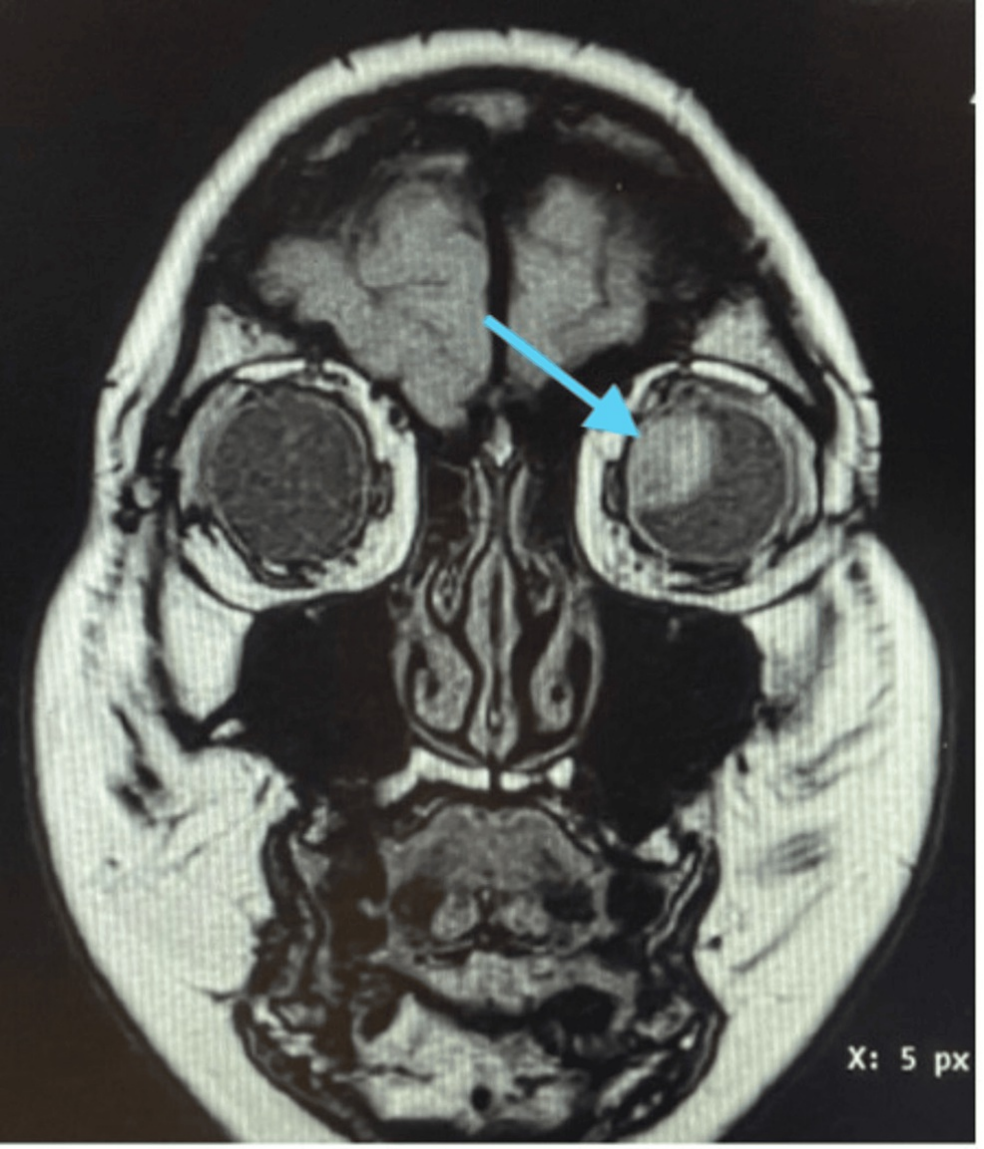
Coronal view T1 post-contrast MRI of the brain and orbits showed an enhancing lentiform-shaped intraocular lesion seen at the superomedial aspect of the left globe (blue arrow)
